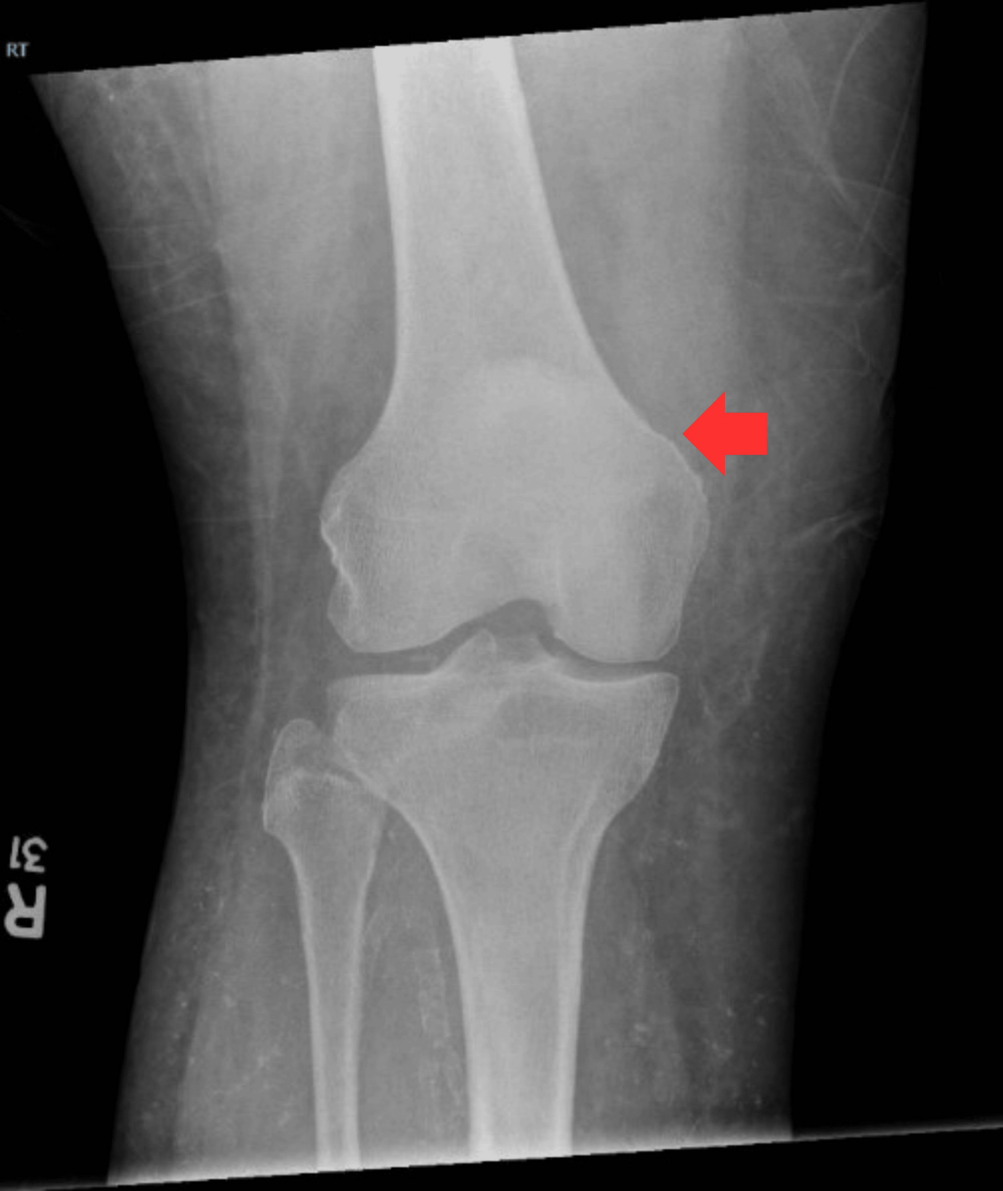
Right Knee X-Ray, Anteroposterior View Anteroposterior view of right knee X-ray taken on initial presentation. There is no abnormality identified in the distal femur or patella on this anteroposterior view. The red arrow points to the patella and distal femur.
